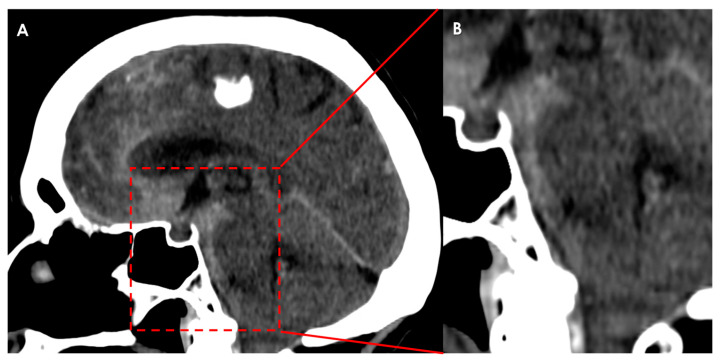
Non contrast CT of the brain (128 rows scanner, slice thickness 3 mm) in a 45-year-old patient with spontaneous non-aneurysmal SAH, reconstructed with multiplanar reconstruction protocol in sagittal plane (A) and magnified detail (B). In both panels, a tiny grayish linear structure is made evident in the prepontine cistern by the natural contrast of the extravasated blood, being highly suspicious for being SLYM.
